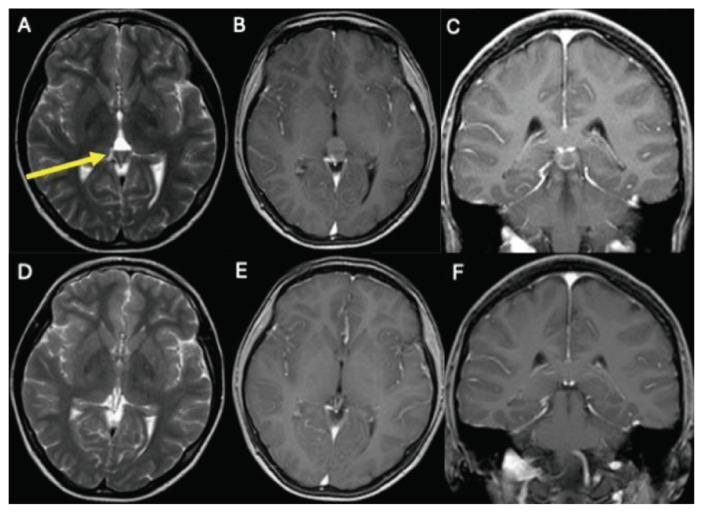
MRI of one patient with a haemorrhaged pineal cyst. A mirror formation is recognisable. (A) Axial T2 sequence with contrast medium before resection. (B) Axial T1 sequence with contrast medium before resection. (C) Coronal T1 sequence with contrast medium before resection. (D) Axial T2 sequence with contrast medium after resection (E) Axial T1 sequence with contrast medium after resection (F) Coronal T1 sequence with contrast medium after resection.
